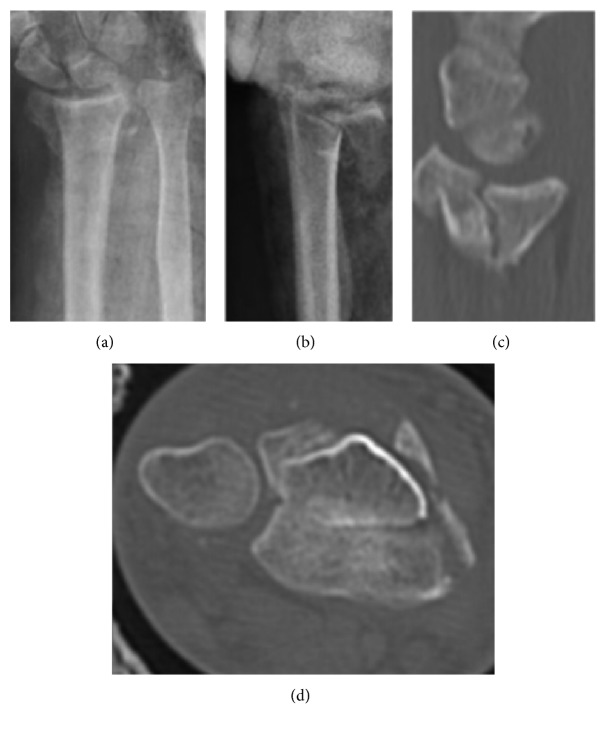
Preoperative X-rays, anteroposterior and lateral view of the 23C2 fracture (a, b). CT scan showing articular damage (c, d).
